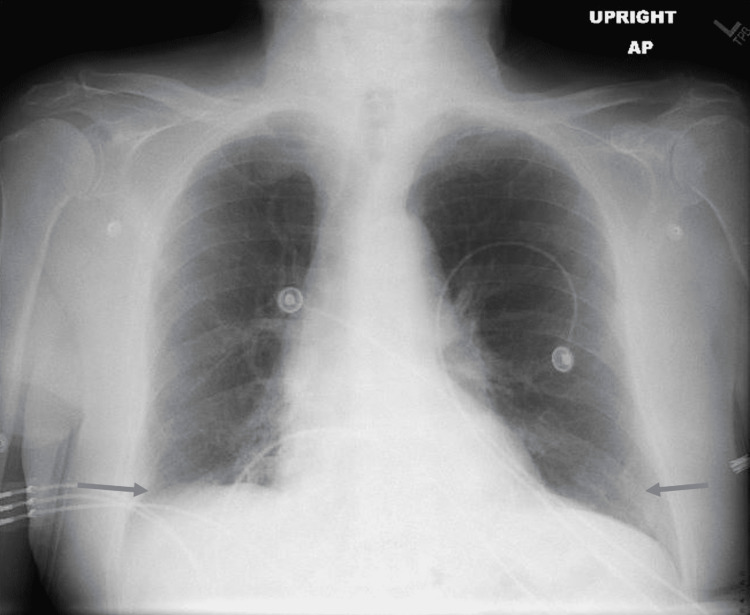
Chest X-ray showing bibasilar opacities
